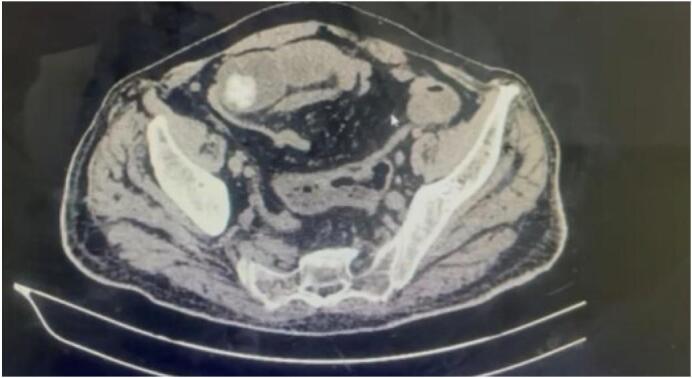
CT scan showing gallstone in terminal ileum.
